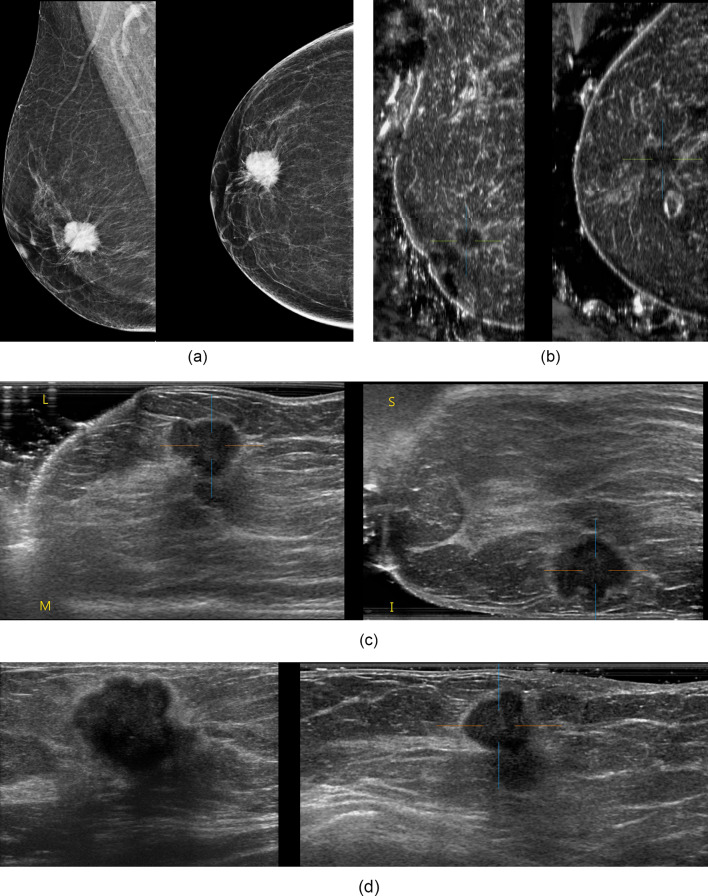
A 58-year-old woman with papillary carcinoma (ER 95% PR 70%, HER2 1+, Ki-67 21.4%). (A) Mammogram shows a mass in the right lower outer quadrant with scattered fibroglanduar (BI-RADS composition grade B). (B) MammouS-N reconstructed MLO (left) and CC (right) image demonstrates a corresponding hypoechoic lesion in the same projection, with clear spatial alignment to the mammographic finding. (C) MLO axial scan (left) and CC axial scan (right) on MammouS-N show irregular hypoechoic mass which is suspicious for malignancy. (D) Hand-held ultrasound (left) and PA view (right) confirm a concordant hypoechoic mass at the same location.
